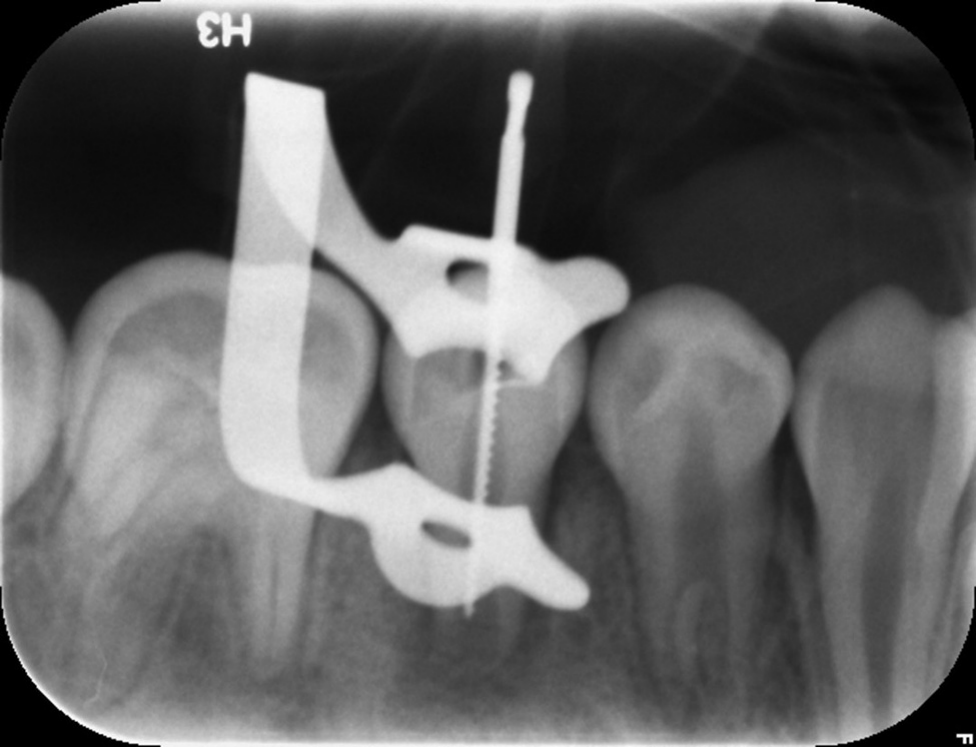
Working length periapical film with #60 K file.
